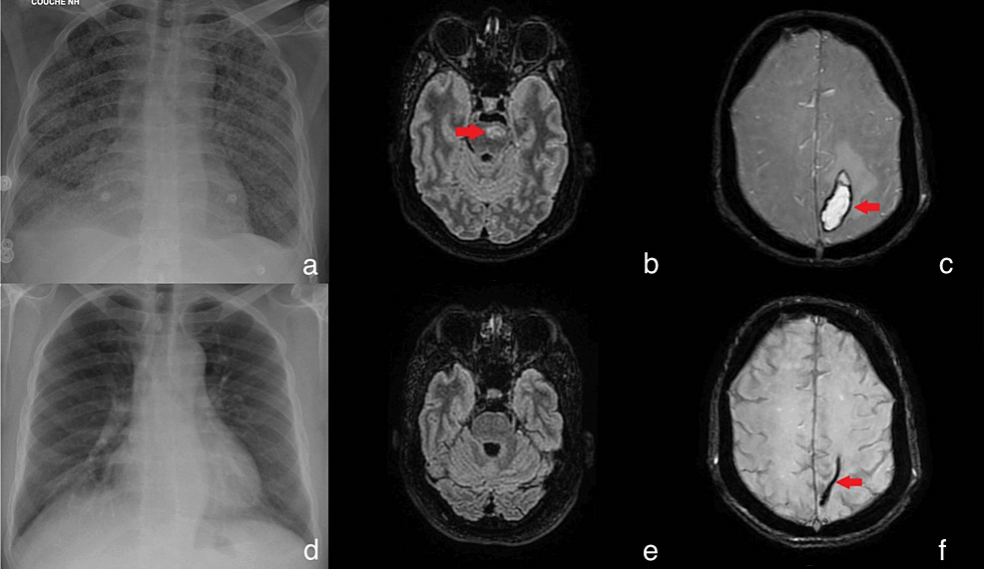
Evolution of intracranial and thoracic findings: a year-long imaging assessment. Imaging at baseline: a. Chest X-ray reveals numerous bilateral small opacities resembling millet seeds. b. Cerebral magnetic resonance imaging displays left median and paramedian pontine lesions. c. Left parietal hematoma observed with perilesional edema. Imaging after a year: d. Resolution of miliary opacity. e. Disappearance of pontine lesions observed. f. Residual sequelae of the parietal hematoma and resolution of its perilesional edema.
